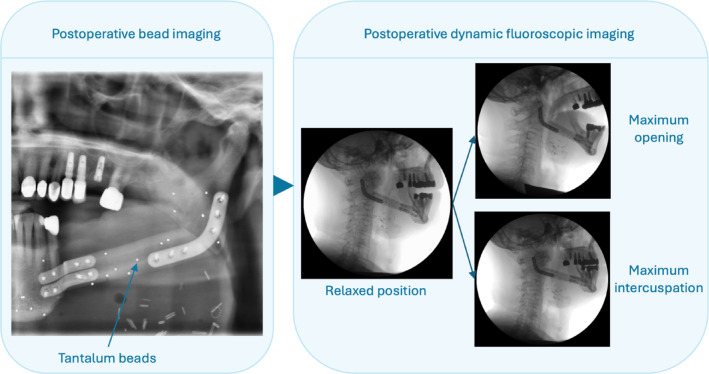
Postoperative and fluoroscopic Imaging. Postoperatively, tantalum beads were identified using routine orthopantomography. After three weeks, fluoroscopy was acquired for maximum opening and maximum intercuspation starting from a relaxed position, respectively.
